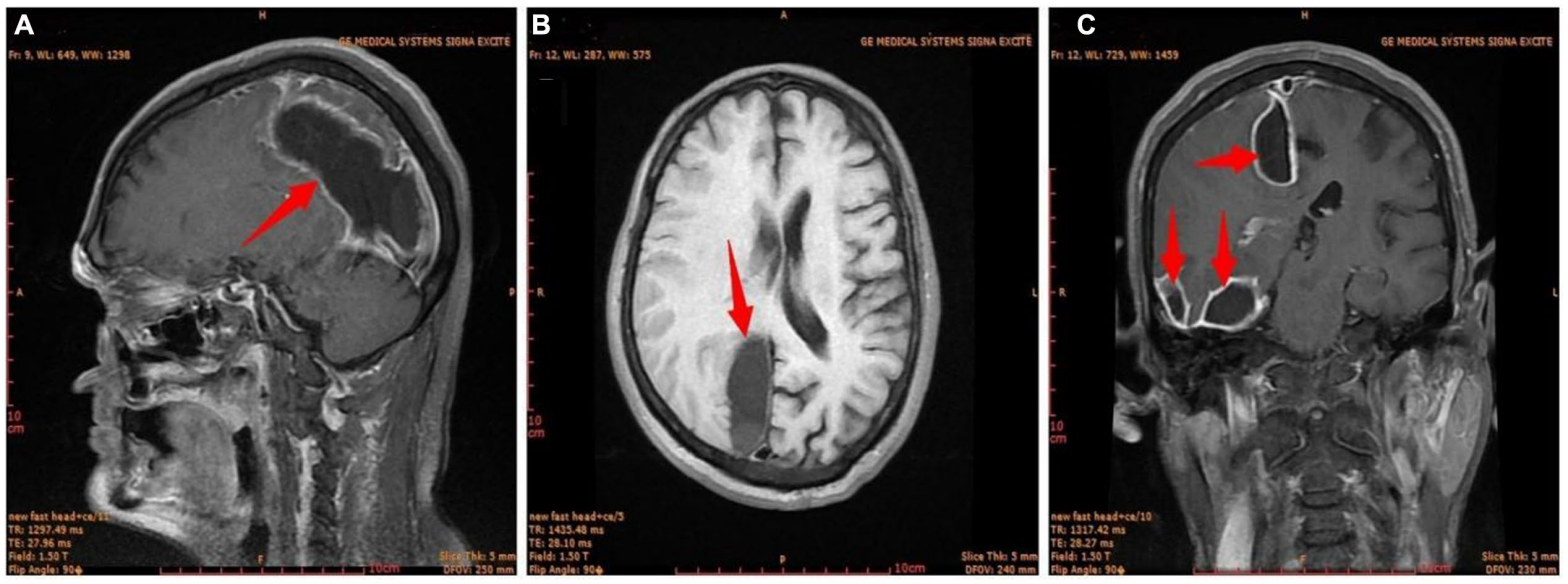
The first postoperative craniocerebral MR image. (A) Sagittal; (B) transverse; (C) coronal. Red arrow, brain abscess.
